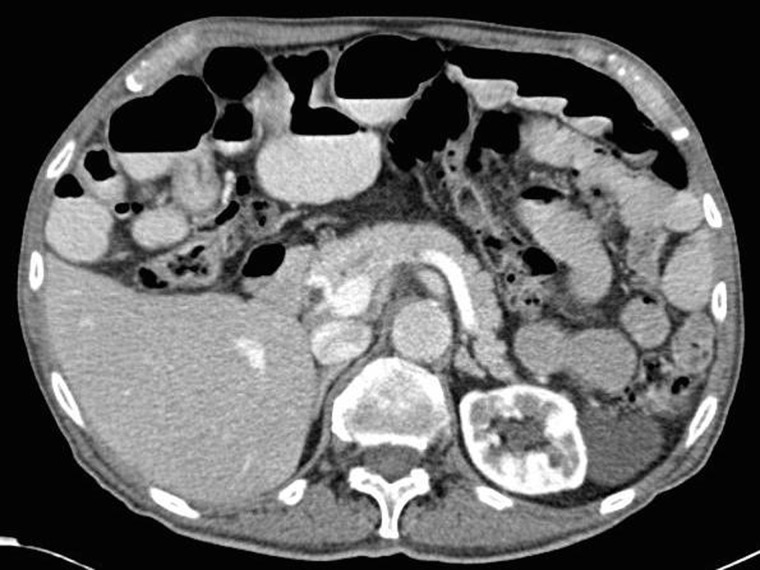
Axial CT scan of the abdomen and pelvis demonstrating extraluminal air between loops of small bowel and diffuse small bowel dilation suggestive of an obstruction.
